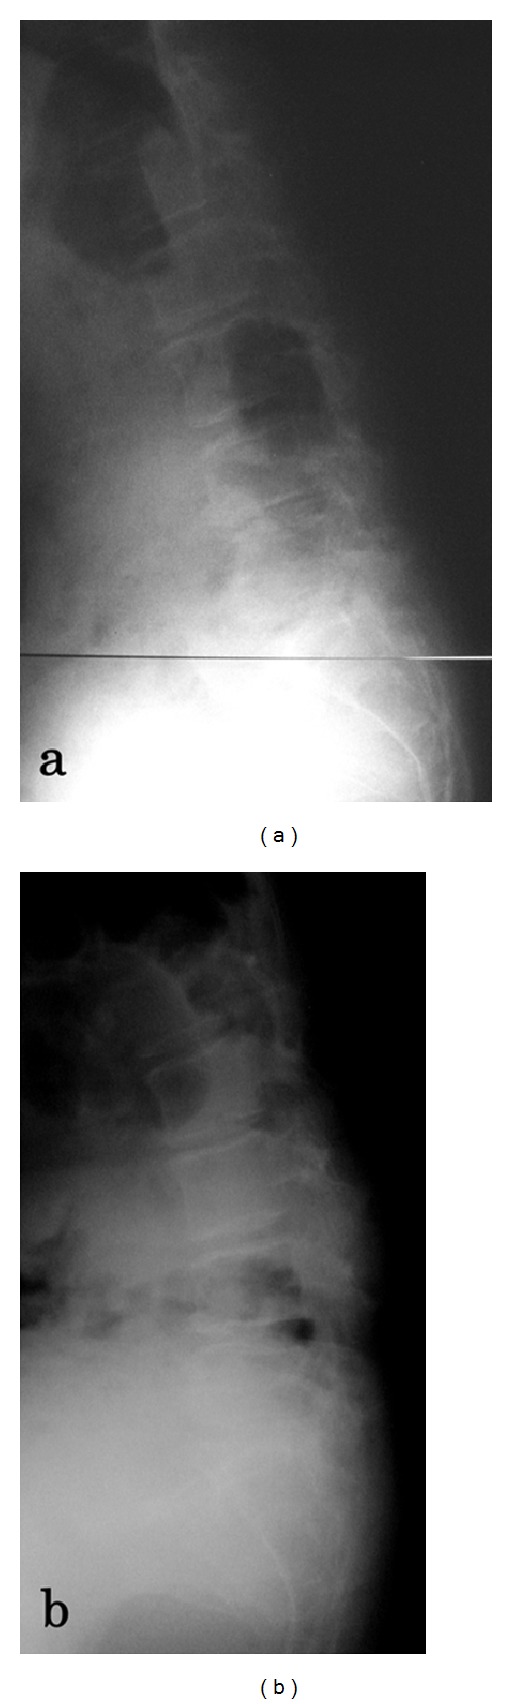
Lateral radiograph of the lumbar spine on lying (a) and standing (b). Kyphosis of the lumbar vertebrae increased on standing.
